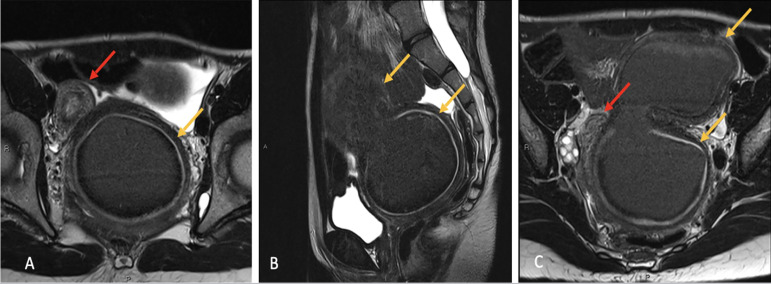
A. Axial T2-weighted MRI image shows a uterus didelphys with two separate
